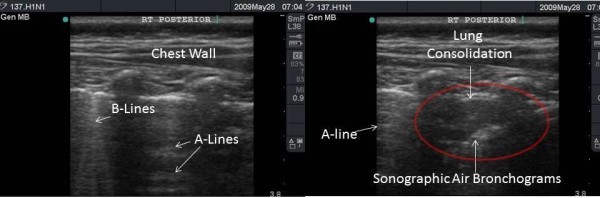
Viral (B-lines) and bacterial pneumonia (lung consolidation with sonographic air bronchogram) pattern. A-lines are horizontal lines that represent the normal aerated lung.
